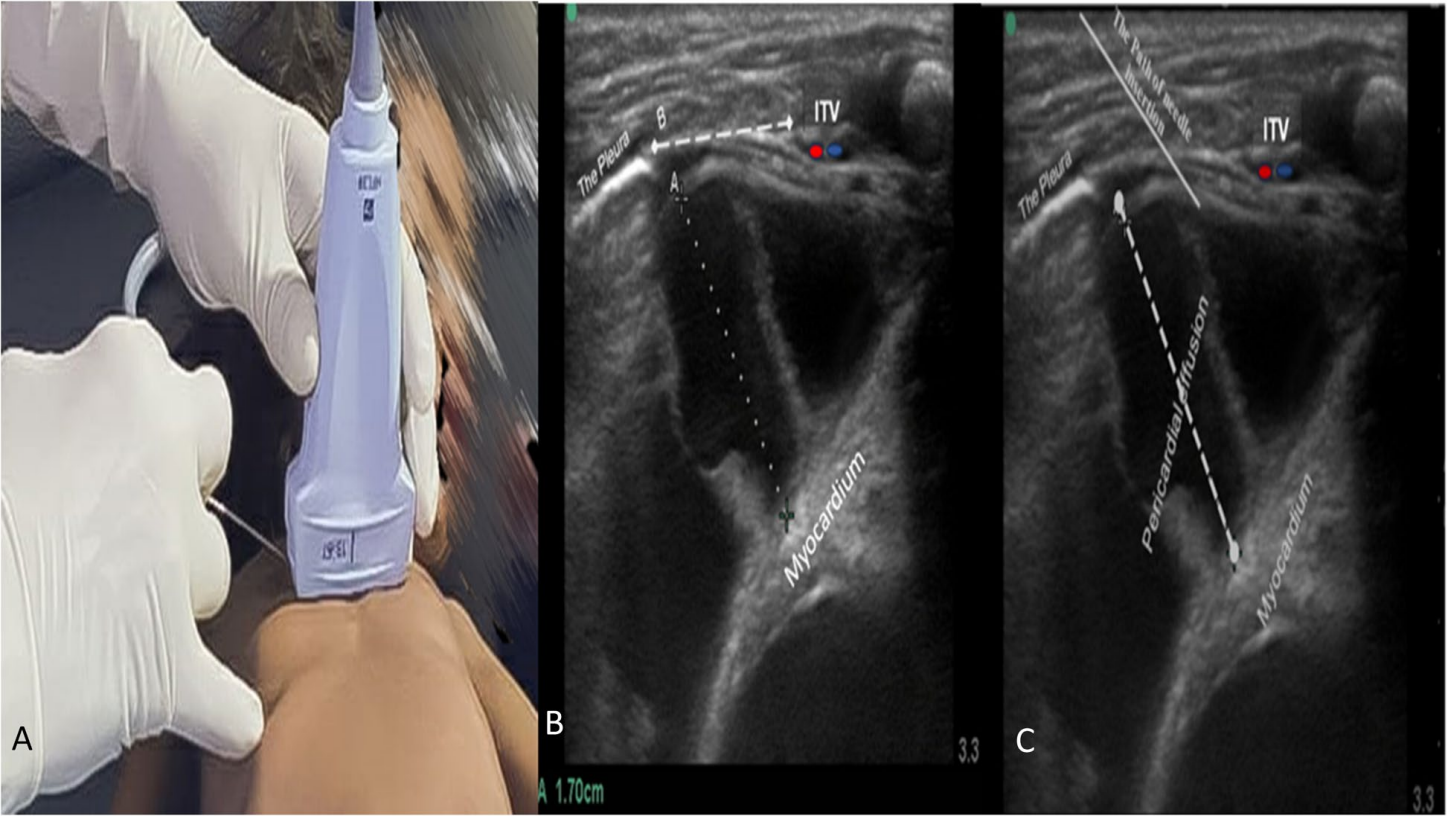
A Image demonstrating the operator handling the high-frequency ultrasound probe and needle insertion using in-plane technique with lateral-to-medial approach at a 45-degree angle. B Parasternal sonogram in long-axis view revealing the cardiac effusion diameter *A* and the distance *B* from the end point of the pleura sliding to the internal thoracic vessels (ITVs). C Parasternal sonographic long-axis view showing the needle’s path of insertion, which passes between the end point of the pleura laterally to the ITVs and medially to the pericardial sac and was visualized in real time during the procedure
